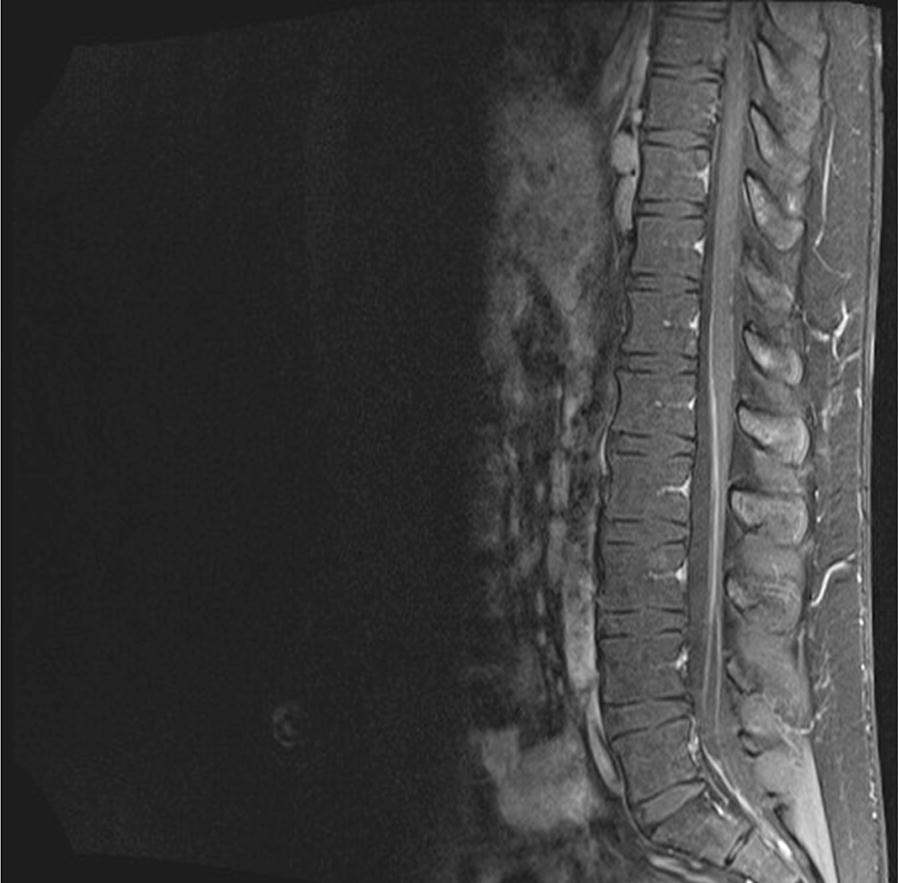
MRI of the cauda equina. Enhancing lesions of the nerve roots are seen consistent with granulomatous inflammation. A biopsy was not possible, given the location
